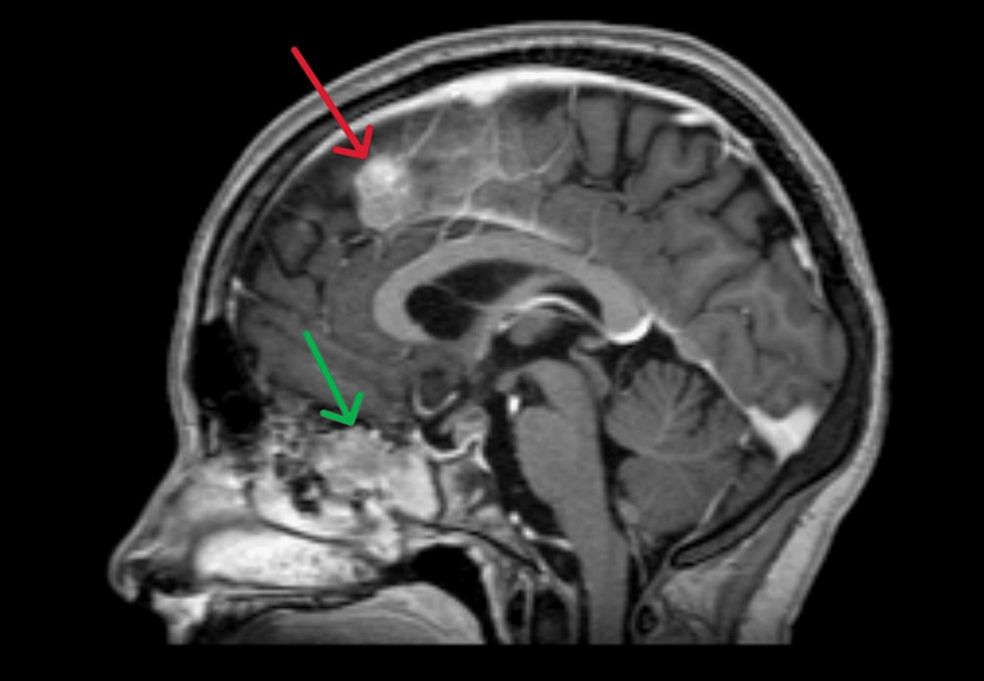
Sagittal T1 MRI with contrast. The red arrow indicates a lesion in a left parasagittal position of the falx cerebri. The green arrow indicates the lesion located in the posterior ethmoidal cells.
